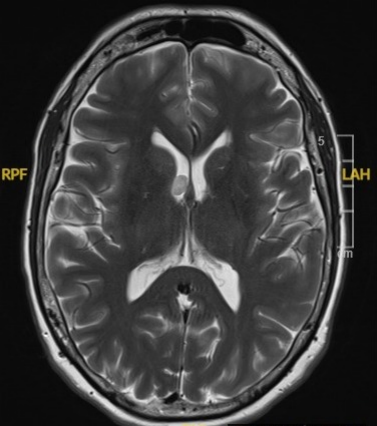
MRI scan (2014) showing lesion right lateral ventricle
